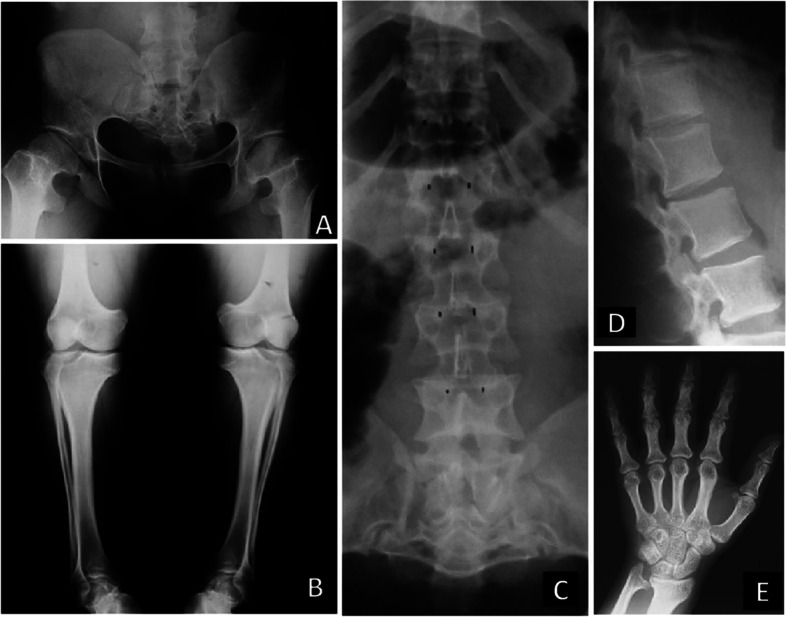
Radiographic images of adults with achondroplasia. A The pelvis shows the same squared shape observed in the early ages, and now the femoral neck is more evident. B In the knees, we can observe the epiphyseal region as broad as metaphysis. Tibiae and fibulae are shorter, however, this last one is longer than the tibiae. C The narrowing of the interpedicular space in the lumbar region is evident in the anteroposterior incidence of the spine X-ray. D In the lateral view of the spine, it can see the concave aspect of the posterior margin of the vertebral bodies. E The hands are short and broad and the ulnar styloid process is prominent
